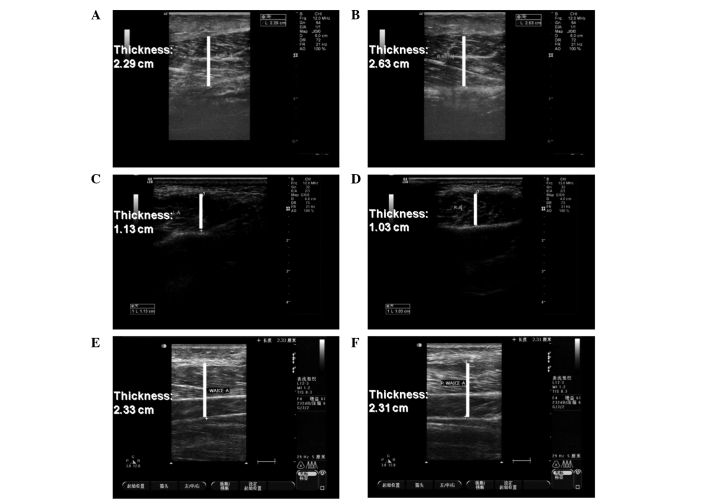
Ultrasound evaluation of the right and left sides of the patient shown in Fig. 2 (A and B) prior to treatment, (C and D) 6 months after treatment and (E and F) 12 months after treatment. The white bar indicates the thickness of the masseter muscle.
